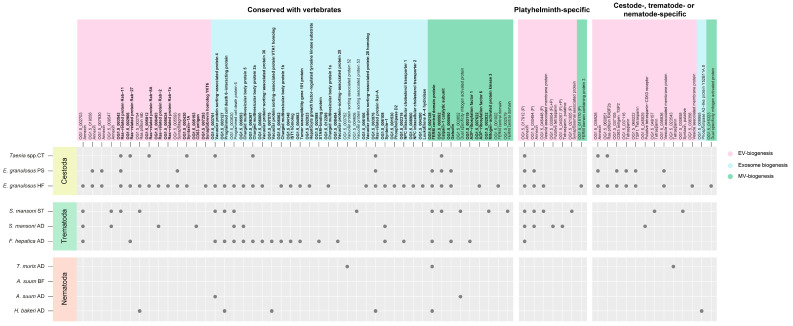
Proteins related to EV-biogenesis detected in EVs from parasitic helminths. Proteins in bold correspond to those described in Bennett et al., 2020 [7]. FL + P: Conserved among free-living and parasitic species; P: Conserved among parasitic species only. Grey dots indicate presence. CT: Cysticercus, PS: Protoscolex, HF: fertile Hydatid Fluid, ST: Schistosomulae, AD: Adult, BF: Body Fluid.
